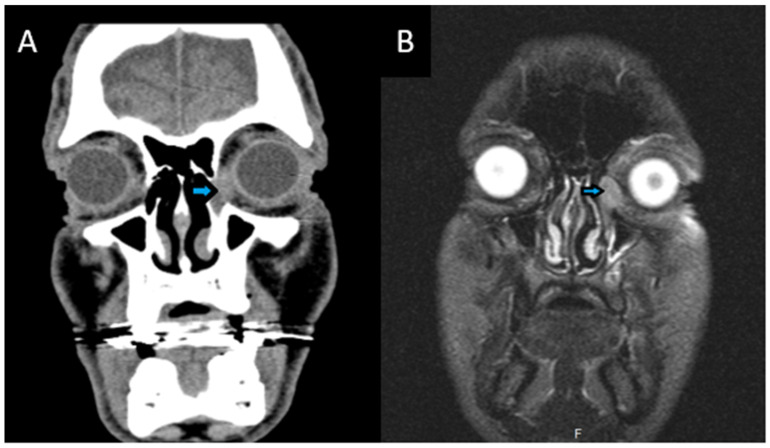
(A) Coronal CT scan of the craniofacial region demonstrating mild swelling (arrow) in the left lacrimal sac region. (B) Coronal T2-weighted MRI of the orbits showing a well-defined mass with high signal (arrow) in the left lacrimal sac.
